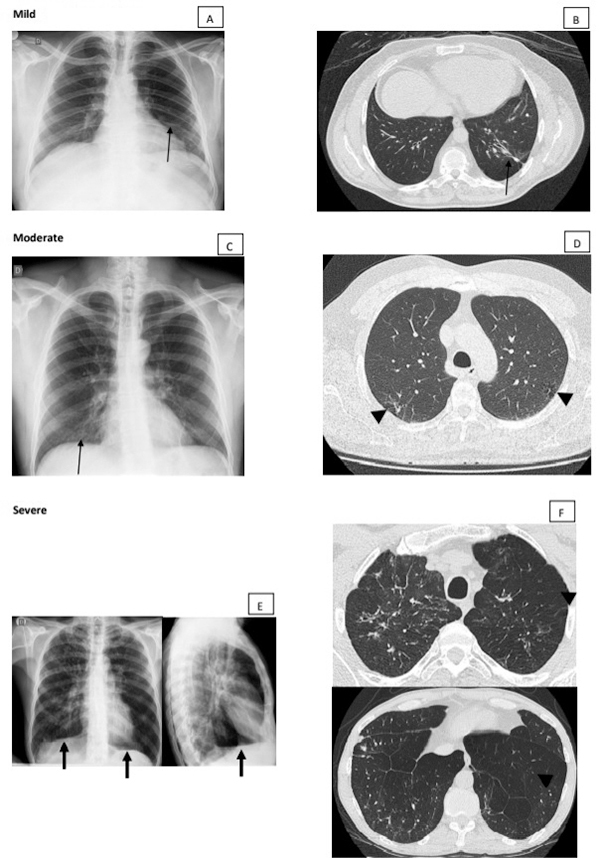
(A, B) Mild residual lesion. Chest radiograph and CT scan of the same patient showing parenchymal band associated with traction bronchiectasis on CT (arrows). Moderate residual lesion: (C) chest radiograph showing intersticial lesions in subhilar regions (arrow) and (D) CT scan showing focal paracicatricial emphysema adjacent to irregular septal thickening (arrowhead). Severe residual lesion: (E) chest radiograph showing interstitial fibrotic lesions and signs of pulmonary hyperinflation, such as low set and flattened diaphragm (arrow). (F) These emphysema signs are better characterized by CT scan (arrowheads).
